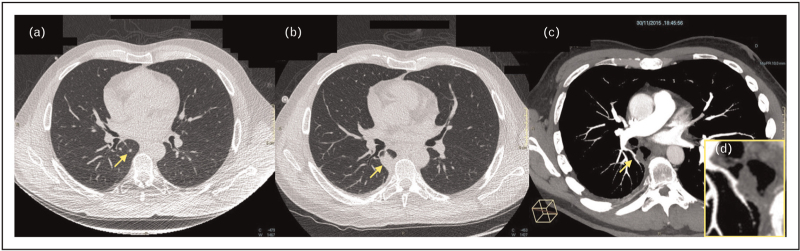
Combined low dose computed tomography (LDCT) imaging with pulmonary angiography to diagnose invasive fungal disease. a 42-year-old man who received alloHCT for acute myeloid leukemia developed Gram-negative *Pseudomonas aeruginosa* sepsis prior to engraftment. Initial LD-CT at the time of initial fever during the first 24 h of neutropenia reveals on axial CT images (a) a small micronodule (<10 mm) in the right lower lung (arrow). Patient developed persistent fever with a repeat LD-CT 10 days later (b) demonstrating enlarged (>10 mm) right lower lobe infiltrate amenable to CTPA pulmonary angiography (arrow). CTPA revealed (c) arterial vessel occlusion (d, inset magnified image) suggestive of angioinvasive fungal disease. Modified with permission from reference [30^▪^]. CT, computed tomography; CTPA, CT pulmonary angiography; HCT, hematopoietic cell transplantation.
